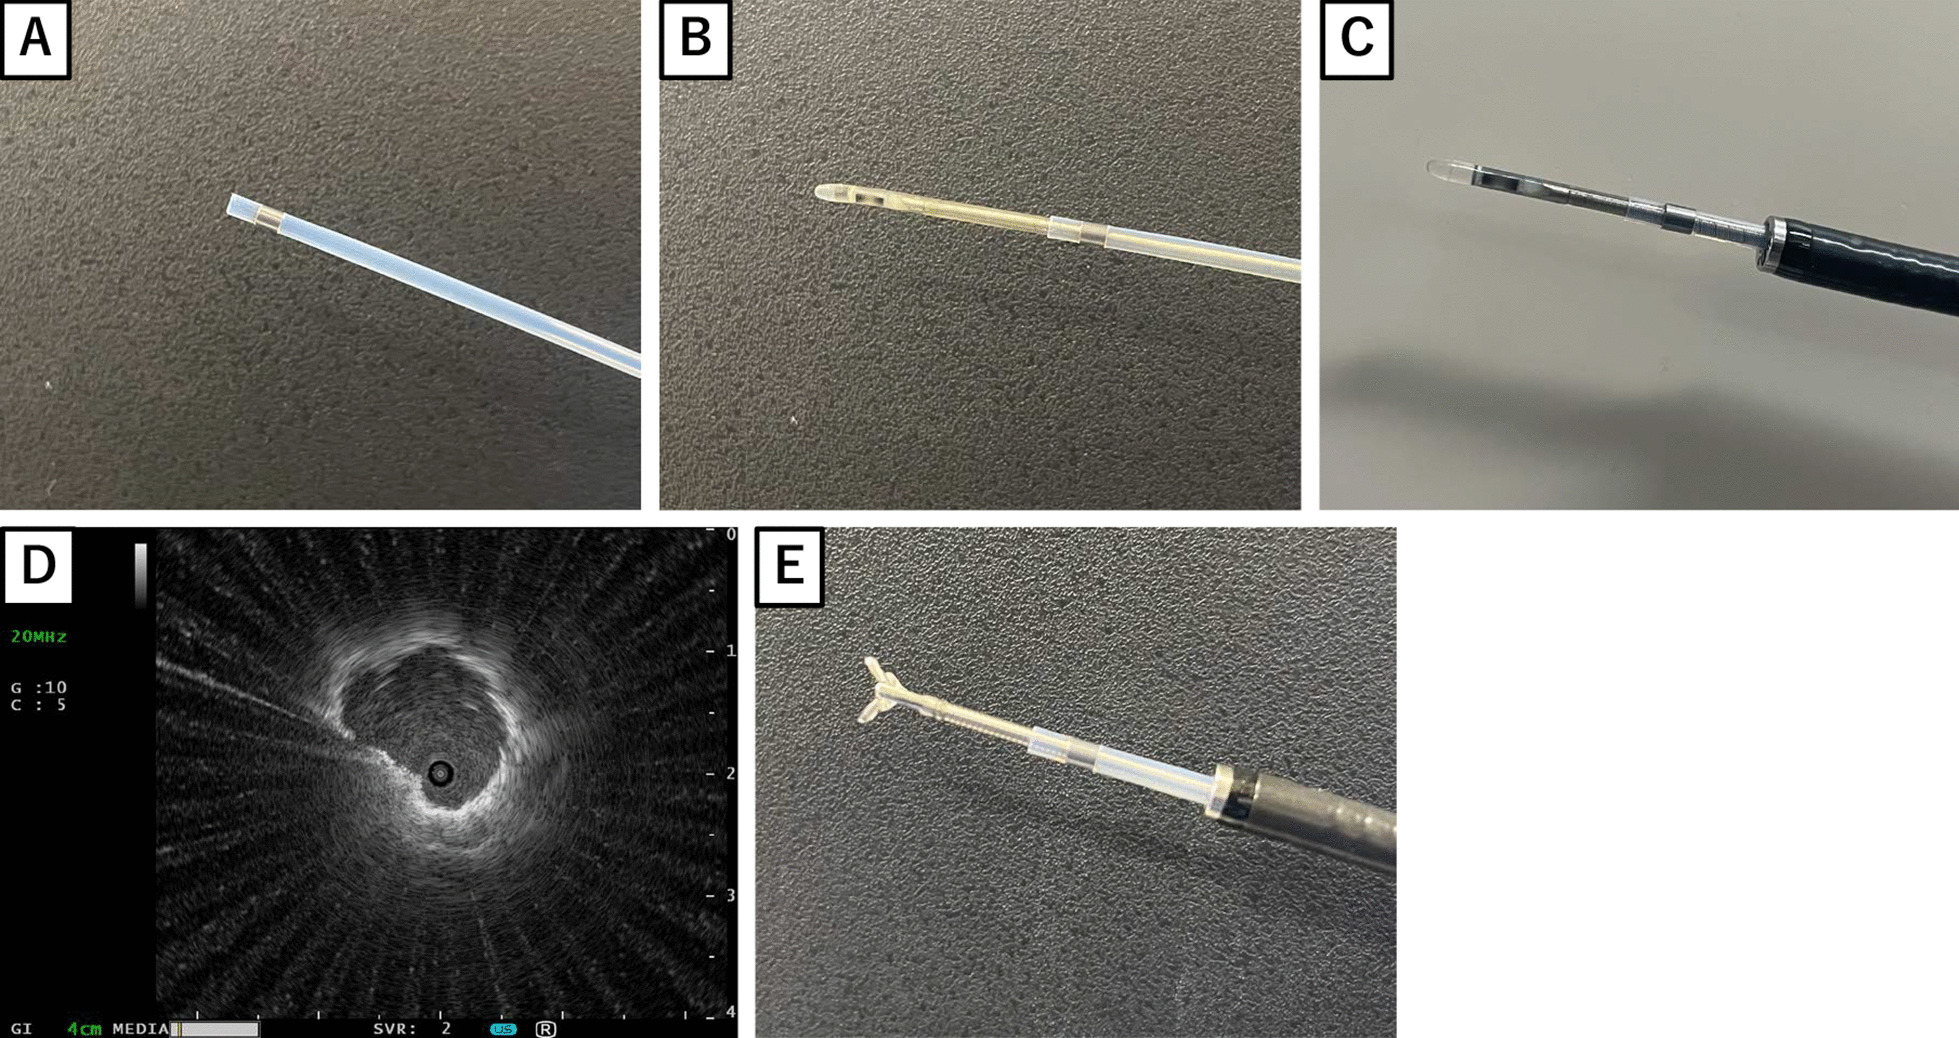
Endobronchial ultrasound (EBUS)-guide-sheath (GS)-guided transbronchial biopsy (TBB). A A picture of the GS. B The GS is attached to the ultrasound probe. C The ultrasound probe with GS is inserted through the working channel of the flexible bronchoscopy toward the target lesion. D The EBUS image is obtained when the ultrasound probe reaches the lesion. E The biopsy forceps are inserted into the GS after removing only the ultrasound probe
